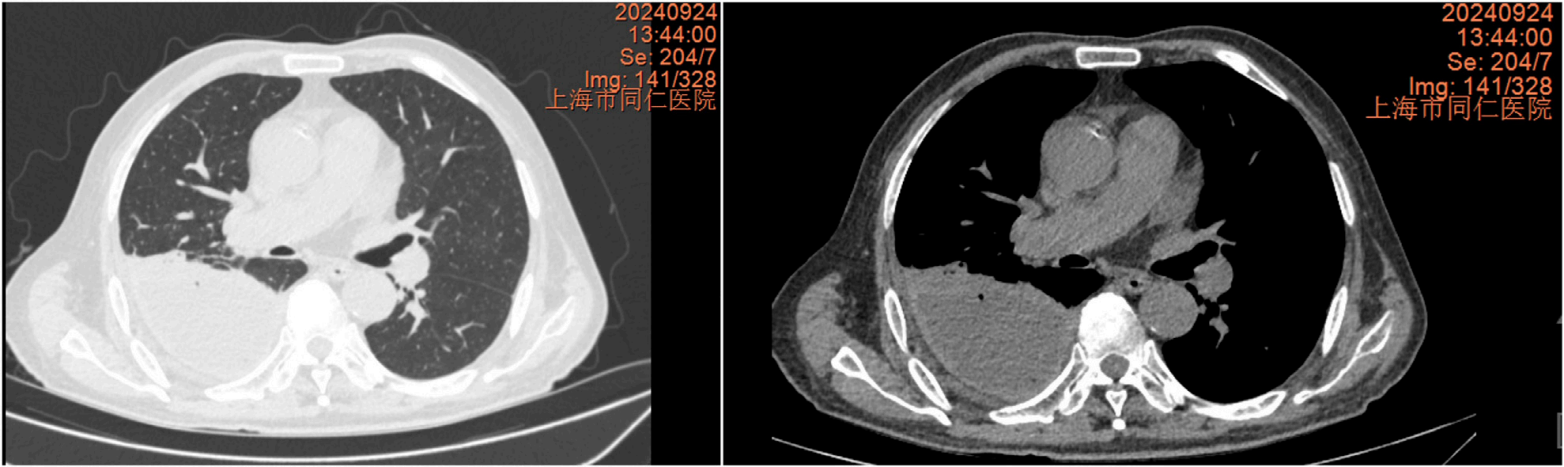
Chest CT scan from 24 September 2024, showing increased pleural effusion, pleural thickening, and bilateral lung inflammation.
